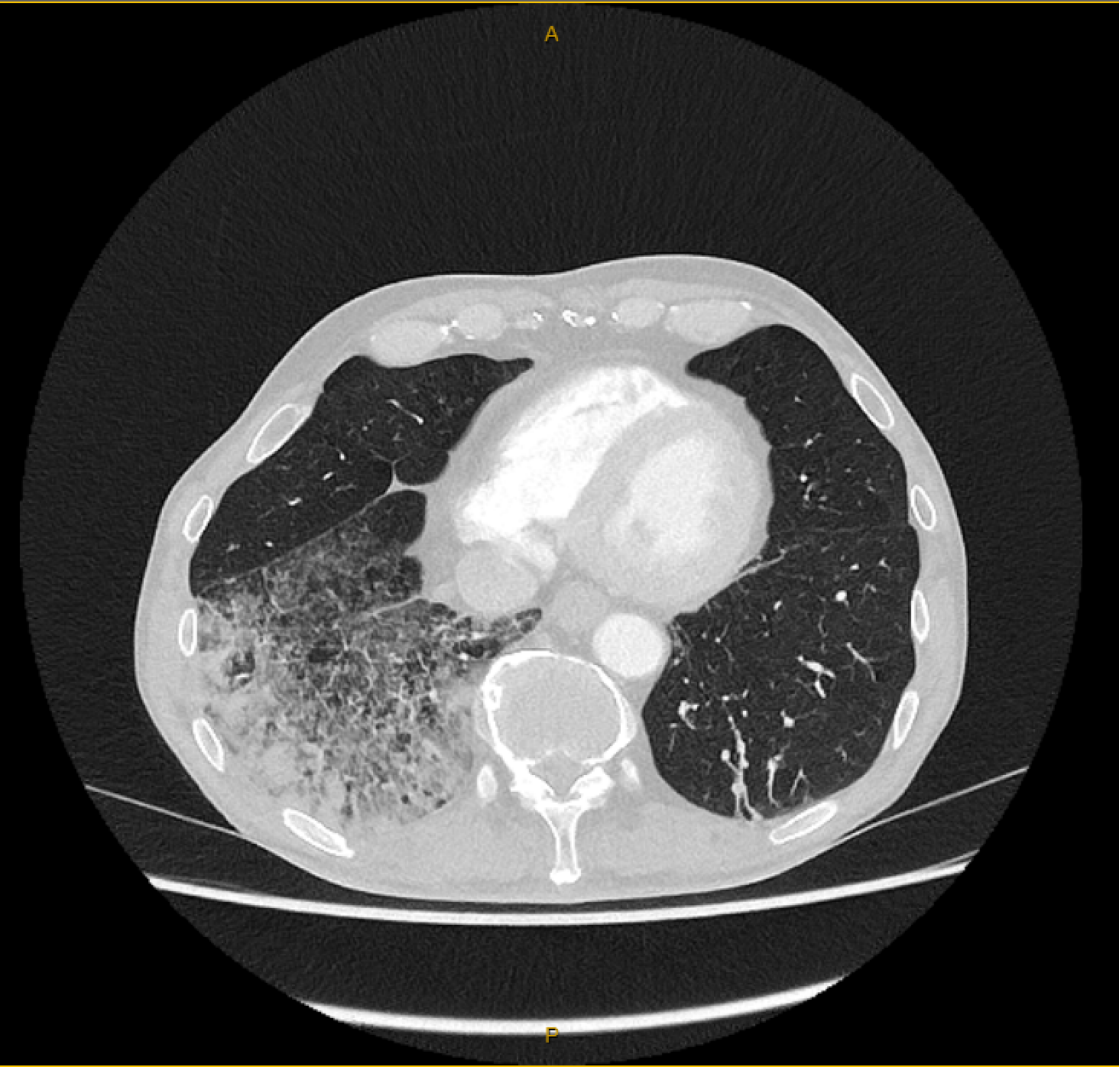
Axial computed tomography (CT) Chest on presentation demonstrating airspace consolidation and right lower lobe reticular shadowing.
